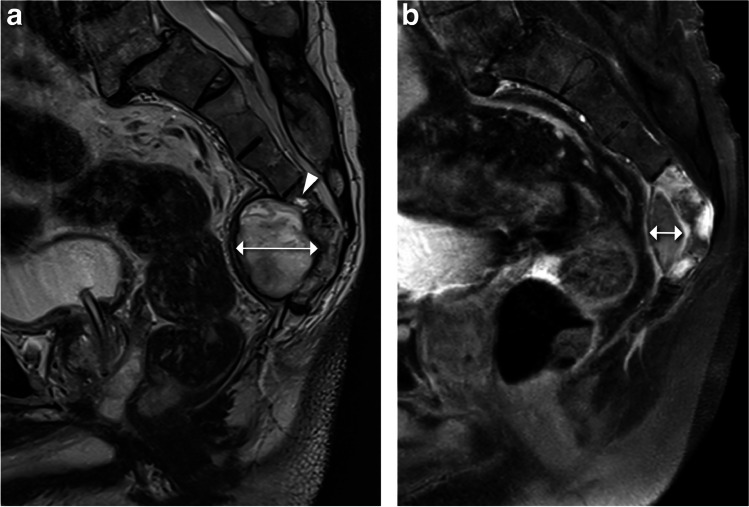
Decrease in size of the tumor’s presacral soft tissue component. a Baseline sagittal T2-weighted MR image shows a focus of hyperintense signal intensity within the marrow of S4 (arrowhead). This finding, alongside the anterior soft-tissue component of the mass, points towards the osseous origin of the tumor. On the baseline MRI, the anteroposterior diameter of the soft tissue mass is 47 mm (double arrow). b Follow-up sagittal contrast-enhanced T1-weighted fat-saturated MR image shows a decrease in size of the anterior soft tissue component (anteroposterior diameter is 16 mm) with rim enhancement and heterogeneous enhancement of intraosseous component
